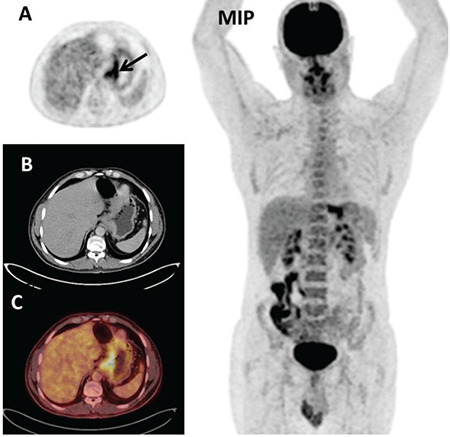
A 48-year-old male patient with SRCC. Axial PET (A), CT (B), and fusion (C) images showed ^18^F-FDG uptake (SUV_max_: 7.9) in primary tumor in the cardia of the stomach (arrow). There was no locoregional lymph node or distant metastasis in PET/CT imaging ^18^F-FDG: Fluorine-18-fluorodeoxyglucose, SUV_max_: Maximum standardized uptake value, MIP: Maximum intensity projection image, PET/CT: Positron emission tomography/computed tomography, SRCC: Signet ring cell carcinoma
